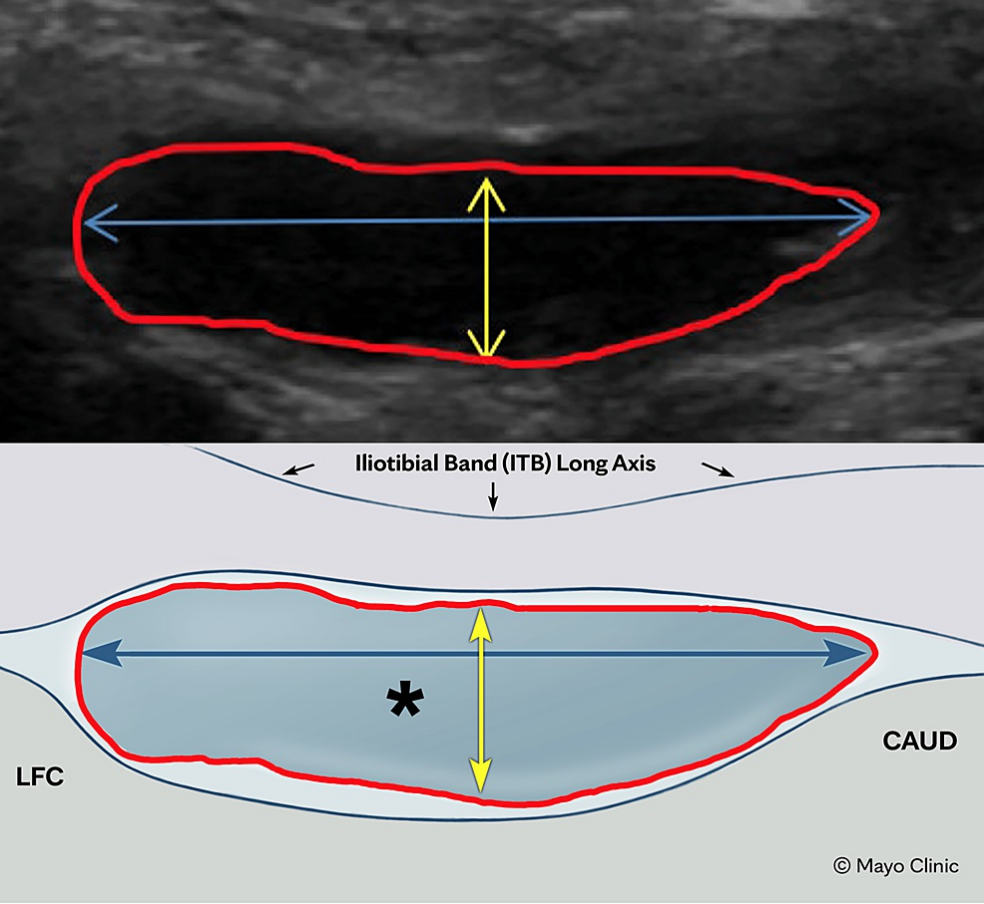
Ultrasound visualization of fluid measurement with representation of structures typically seen. Blue arrow (horizontal), long axis for area calculation by ultrasound machine (LAA).
